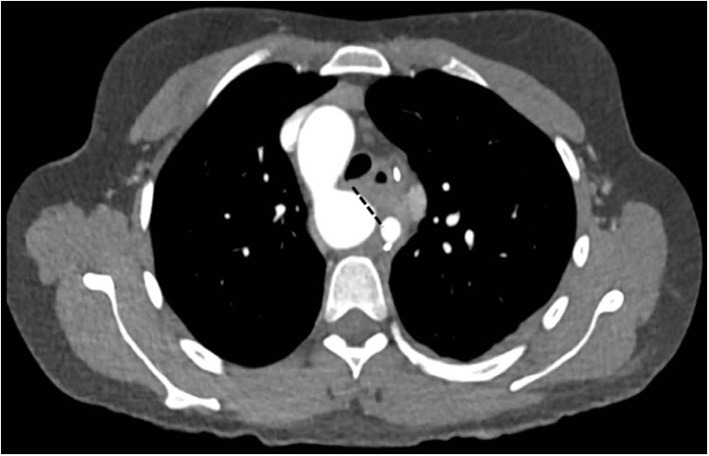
Postoperative CT-angiography after resection of the diverticulum (dotted line). Just on the right is the distally translocated left subclavian artery. Reproduced with permission from Cardiovasc Med: w10132: doi 104414.
